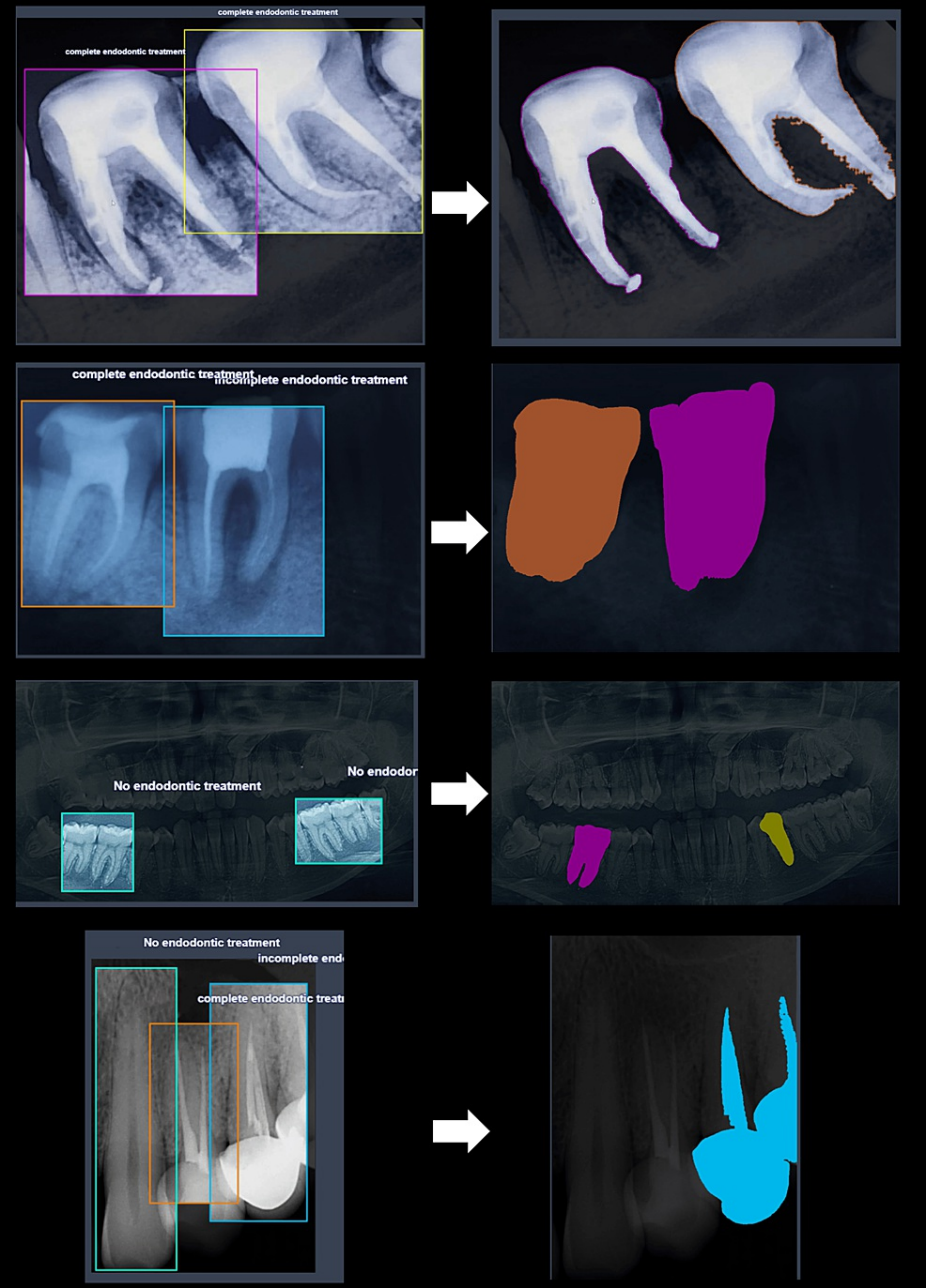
Bounding boxes outlining areas of interest (left) on dental radiographs and the predicted masks for segmentation (right) as derived from CAST outputs CAST: clinical annotation and segmentation tool
